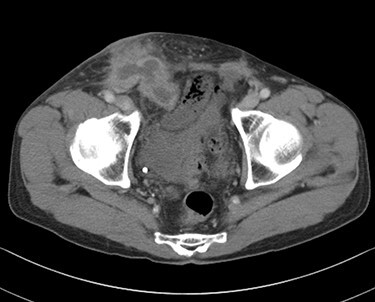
Computed tomography scan revealed abscess formation with contrast effect in the right inguinal area.
